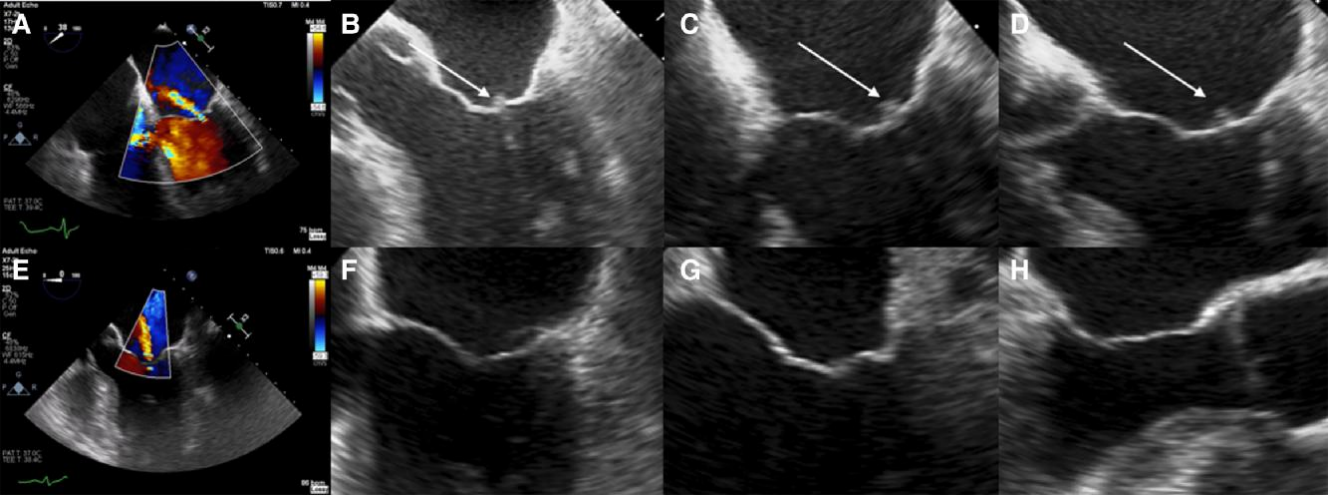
Transoesophageal echocardiogram before (*A–D*) and after treatment (*E–H*). (*A*) Colour flow Doppler showing +2 mitral regurgitation. (*B*) 0° mid-oesophageal two-chamber view of the mitral valve showing a 4 mm vegetation on the posterior and anterior leaflet tips. (*C* and *D*) 38° and 20° mid-oesophageal two-chamber views pre-treatment. (*E*) Colour flow Doppler showing residual 2+ mitral regurgitation. (*F*) 0° mid-oesophageal two-chamber view of the mitral valve showing complete resolution of the vegetation on the posterior leaflet tip. (*G*) 30° mid-oesophageal two-chamber view and (*H*) 128° mid-oesophageal AV long axis view of the mitral valve post-treatment.
